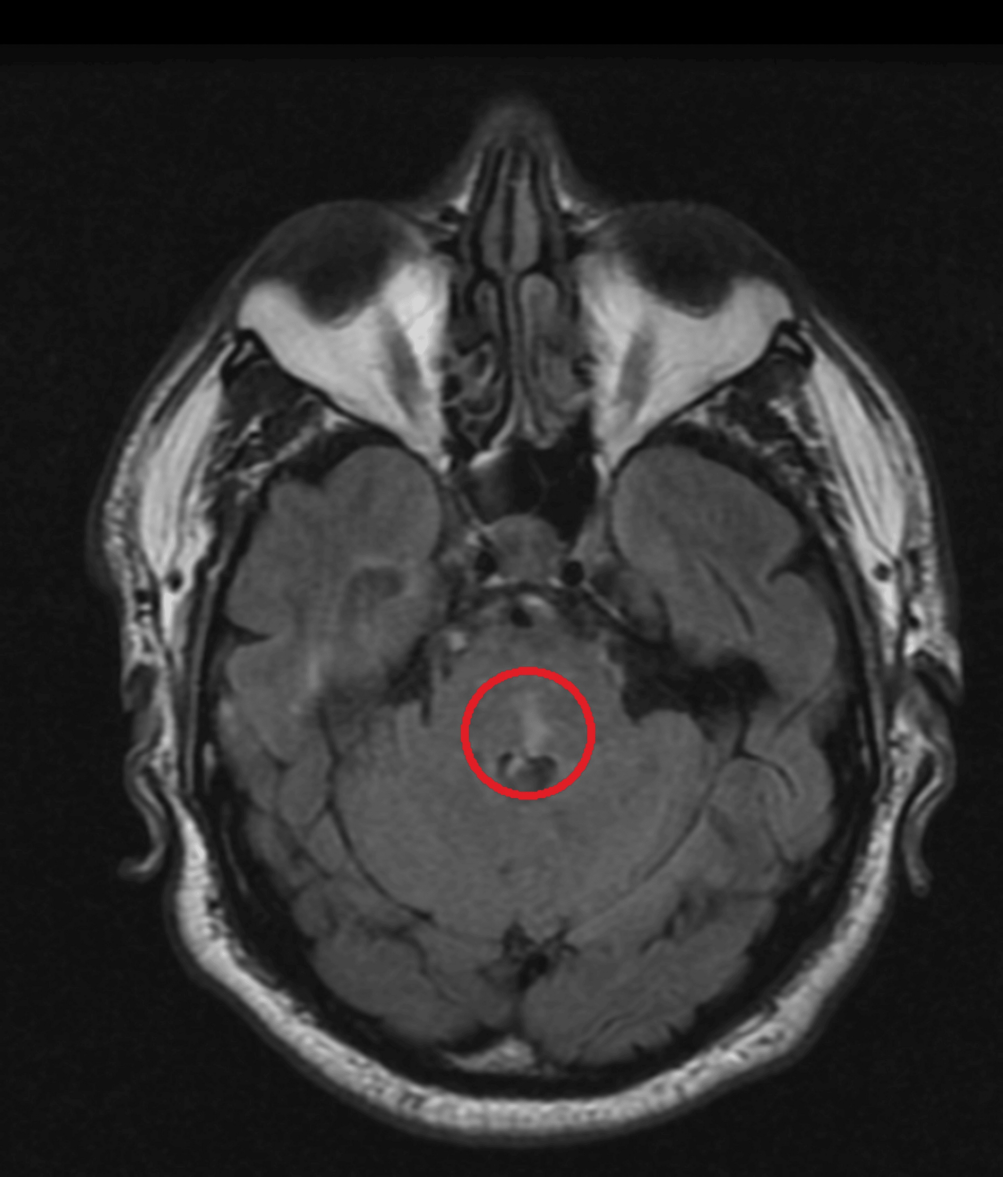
Axial view of T2 brain MRI revealed para-sagittal, non-enhancing, restricted hyperintense focus in the posterior aspect of the left side of the pons, indicating the early stage of a subacute infarct MRI: magnetic resonance imaging
